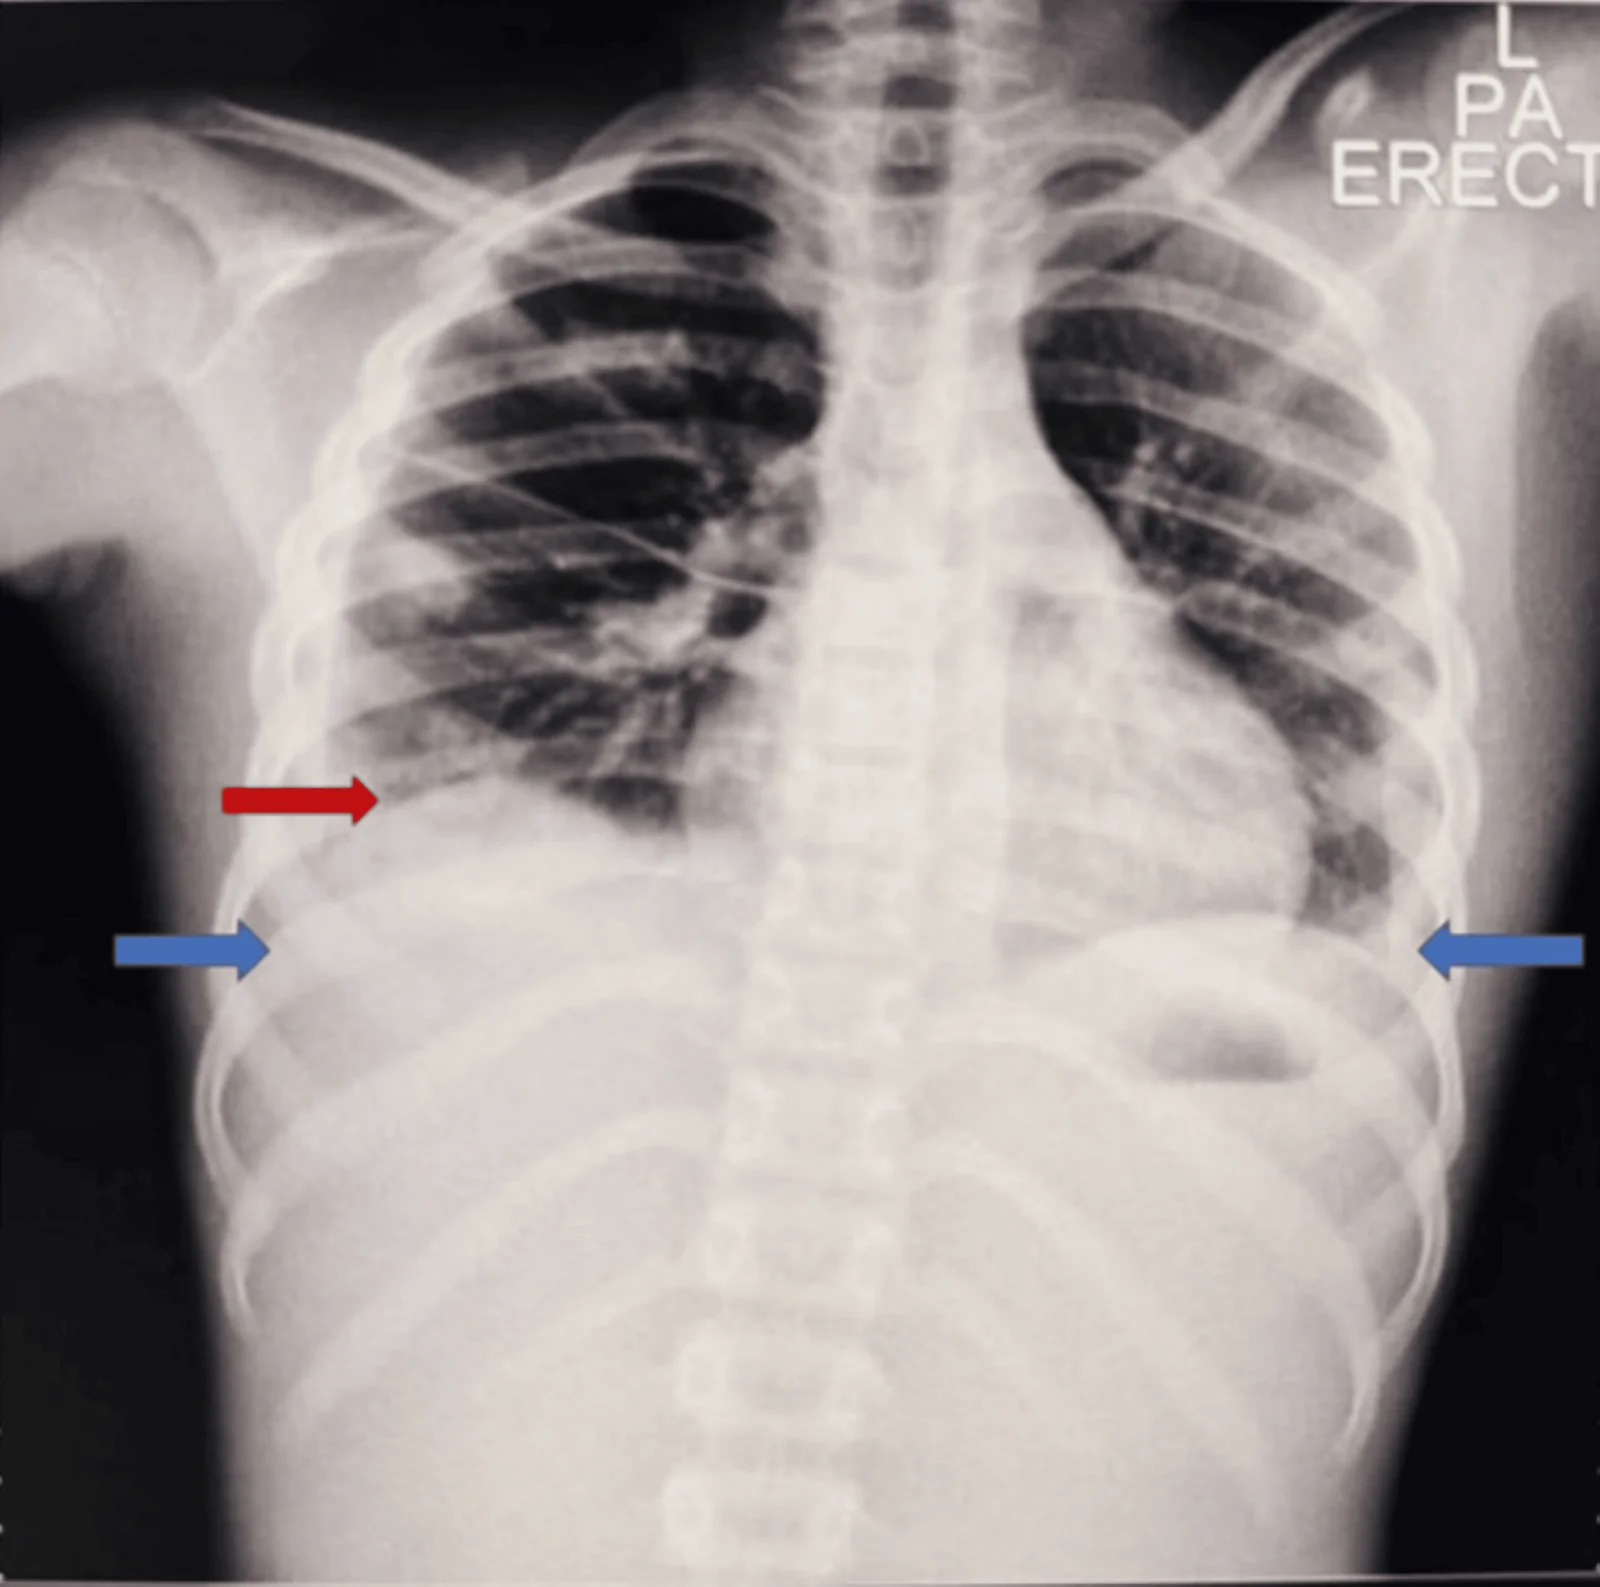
Chest radiograph showing bilateral pleural effusions (blue arrow) and right basal consolidation (red arrow), consistent with pneumonia or subsegmental atelectatic change.
